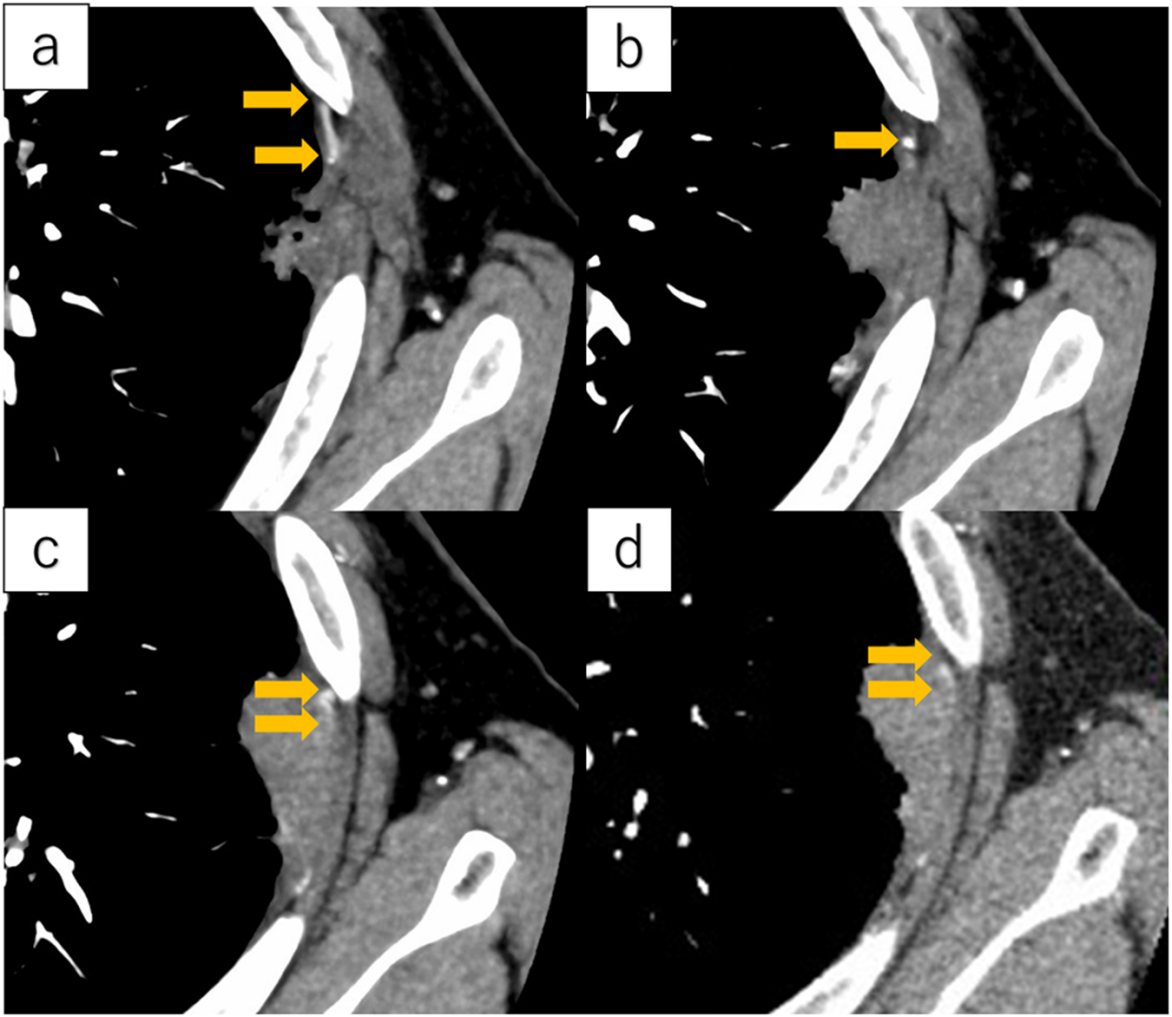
Ultra-high-resolution CT images of a 73-year-old male showing squamous cell carcinoma infiltrating the chest wall in the left upper lobe. a, b, and c are axial sections arranged from bottom to top. d is a conventional HRCT image. The tumor diameter was 29 mm and showed chest wall vessel involvement in subpleural lung cancer (arrow). The postoperative pathology assessment confirmed parietal pleural invasion of the tumor
